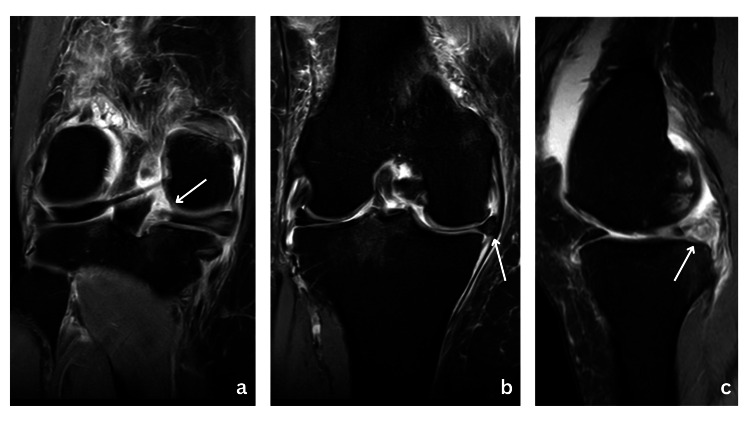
(a) Coronal MRI showing a tear at the medial meniscus root insertion (white arrow). (b) Coronal MRI highlighting extrusion of the medial meniscus, consistent with a root tear (white arrow). (c) Sagittal MRI clearly depicting a "ghost sign," nonvisualization of medial meniscus posterior root insertion (white arrow).
